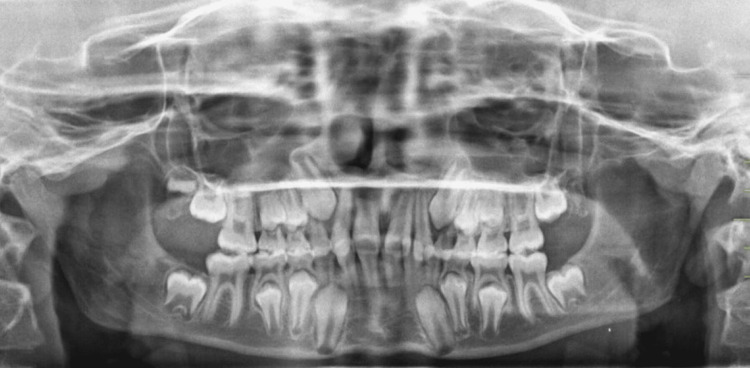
Pre-treatment orthopantomogram (OPG)
